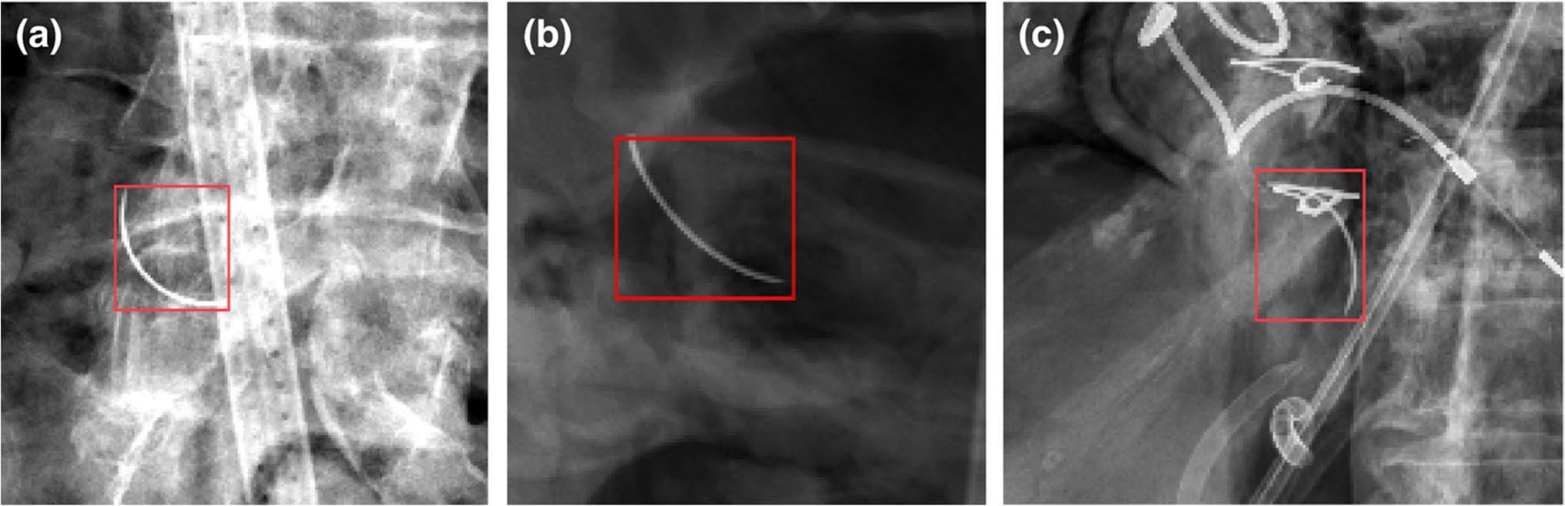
Needles of various shapes and orientations with different backgrounds that were detected by the CAD system. Figure reproduced with permission from Sengupta A, Hadjiiski L, Chan HP, Cha K, Chronis N, Marentis TC. Computer-aided detection of retained surgical needles from postoperative radiographs. Med Phys. 2017;44(1):180–191.10.1002/mp.12011
